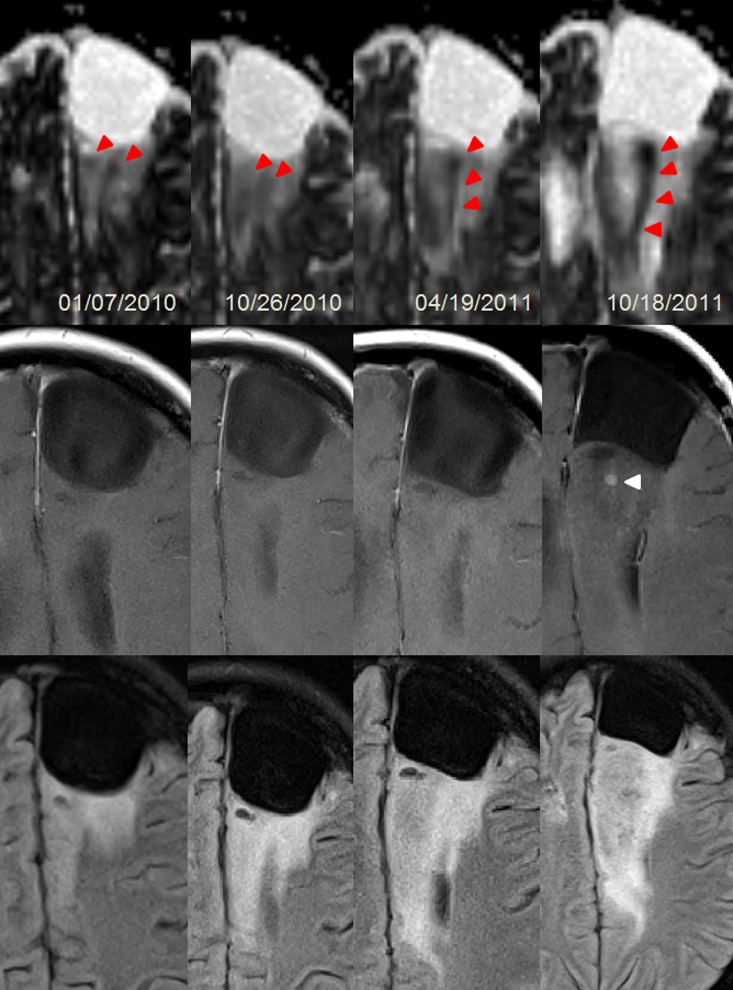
Female patient (24y) with a malignant transformation (anaplastic astrocytoma WHO III) of a primary fibrillary Astrocytoma WHO II. The transformation according to currently accepted radiologic definition was visible as a new punctual CE at the last column. However, changes in cellularity (red triangles) were preceding CE (white triangle) more than one year. The area of the most focal diffusion restriction corresponds to the focal uptake. Upper row: Axial diffusivity maps; Middle row: T1w-CE; Bottom row: T2w-FLAIR. Dates are given in day/month/year.
